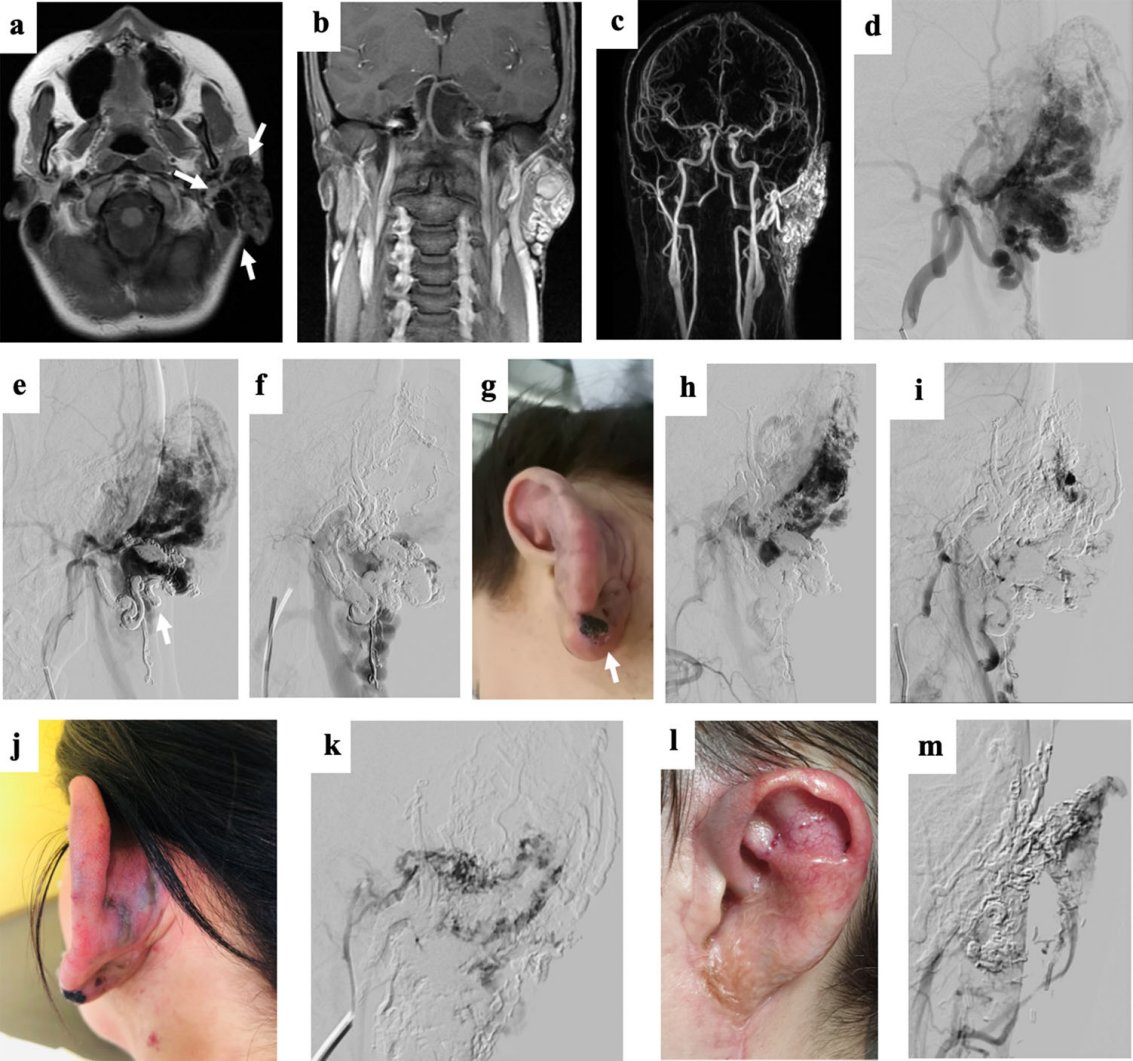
30-year old female patient presenting with an extensive arteriovenous malformation (AVM) left (peri)auricular. After 4 sessions of embolotherapies with corresponding near-total devascularization of the malformation, previously planned two-step microsurgical resection and defect reconstruction using a free fascia flap was performed. (a–c) Preprocedural axial T1-weighted (T1-w) MR image, coronar T1-w MR image, and coronar MR-angiography image present the extent of the left (peri)auricular AVM with ubiquitous involvement of the auricle (arrows) and at least 4 arterial feeders (2 × from facial artery, 2 × from posterior auricular artery, 1 × from occipital artery) as well as venous drainage into the external jugular vein. (d–e) Periprocedural digital subtraction angiography (DSA) images during 1st embolotherapy show flow characteristics of the lesion and the successfully embolized vascular structures at the caudal part of the earlobe (*arrow*). (f) Periprocedural DSA image during 2nd embolotherapy shows the newly embolized vascularized components of the malformation. (g) Clinical photograph 1 day before the 3rd embolotherapy presents the enlarged and prominent ear auricle and lobule, transparent vascular structures of the malformation, and a small, long-term necrotic area on the caudal ear lobe. (h + i) Periprocedural DSA images during 3rd embolotherapy demonstrate remaining vascularized components of the malformation (*arrow*) as well as successfully embolized cranial components of the lesion. The patient presented with visual disorders directly post-procedural and MRI revealed 2 subtle restricted-diffusion lesions including the visual cortex, most likely due to periprocedural small air emboli. These findings were entirely regressive while preventive monitoring at the stroke unit. (j) Clinical photograph 3 days after the 3rd embolotherapy. (k) Periprocedural DSA image during the 4th embolotherapy shows arterial bleeding occurred after minor manipulation under anesthesia, due to previously unnoticed secondary abscessing after the 3rd session. After abscess draining and embolization of the arterial bleeding using histoacryl/lipiodol mixture, Onyx embolization of the remaining AVM components was successfully performed in the same session. (l) Clinical photograph after initially planned surgical resection of the devascularized AVM tissue including parts of the ear auricle and lobe and defect reconstruction with means of a free serratus fascia flap both successfully performed 3 months after the 4th session. (m) Diagnostic DSA image at final follow-up (21 months after the last treatment) presents a good perfusion of the apical ear auricle as well as no novel AVM components. Clinically, the patient appeared without any symptoms or further signs of AVM recurrence
